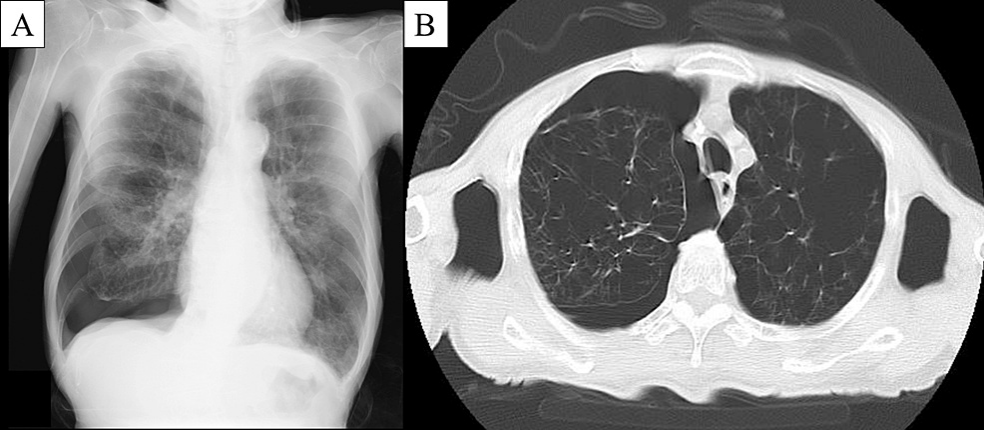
Chest radiography and computed tomography before chest tube insertion Chest radiography (A) and computed tomography (B) reveal a right pneumothorax in a patient with severe emphysema, and moderate adhesions between the lung and chest wall.
